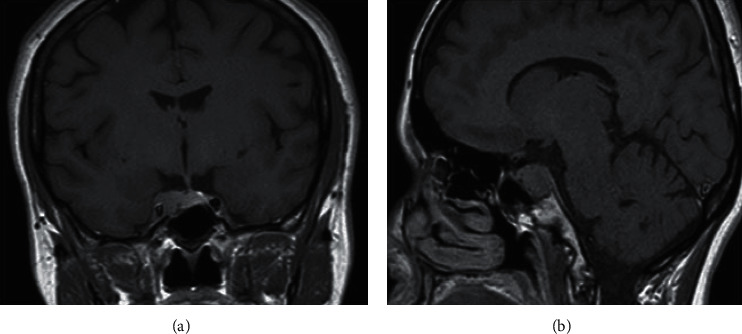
Coronal (a) and sagittal (b) images of pituitary MRI showing 10 mm hypoenhancing adenoma with invasion of the right cavernous sinus.
